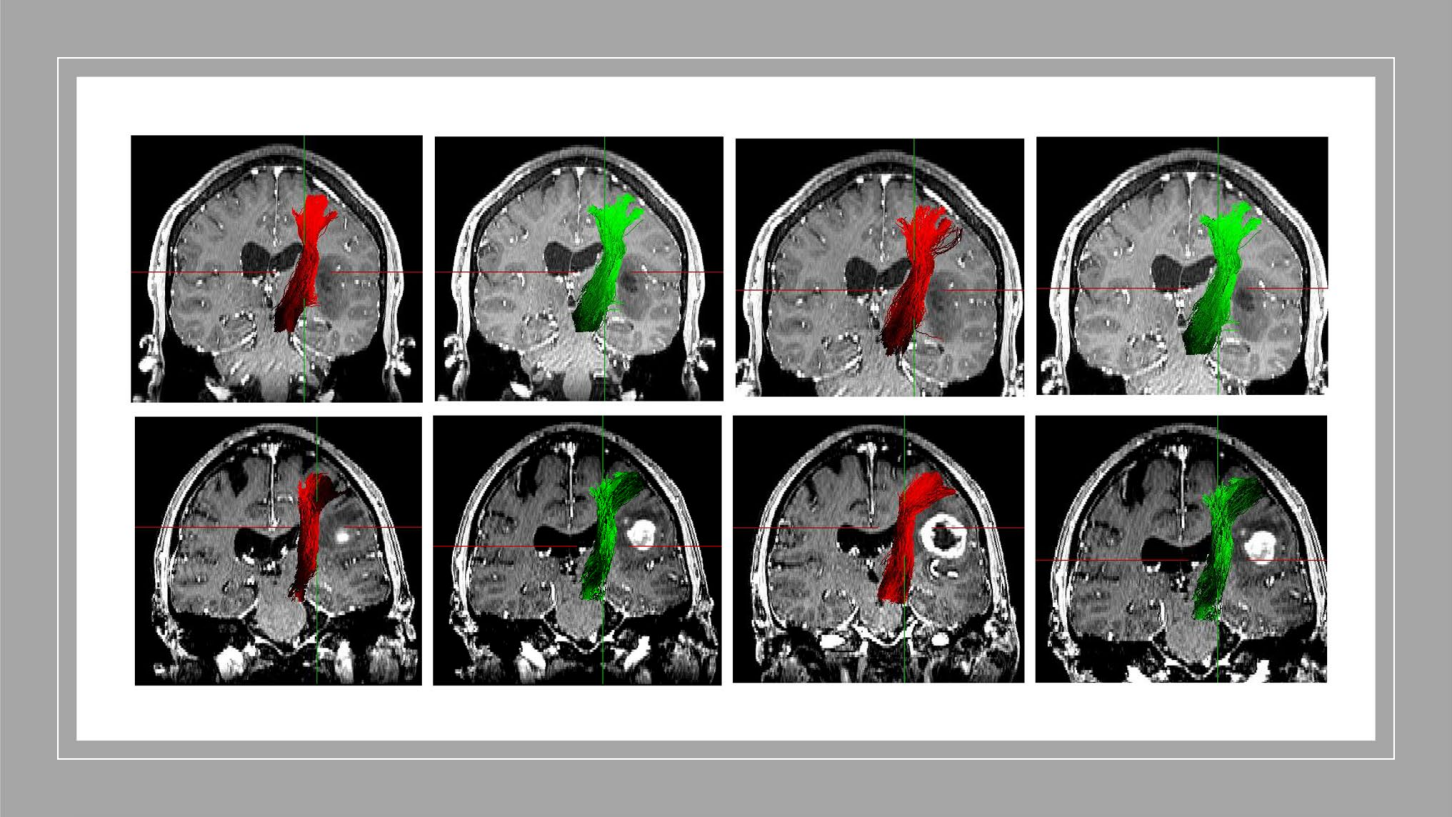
MRI T1-weighted + gadolinium in axial oblique view displaying a non-contrast enhancing temporo-occipital glioma in the proximity of the OR. From left to right: Rater 1, DTI-FT, Rater 1, QBI-FT. Rater 2, DTI-FT, Rater 2, QBI-FT. (MRI, magnetic resonance imaging; OR, optic radiation; QBI, Q-ball Imaging; DTI, Diffusion Tensor Imaging)
